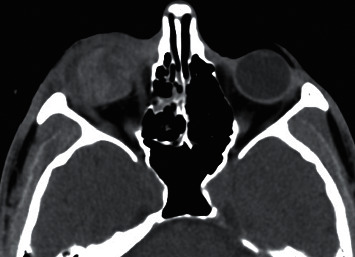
Open globe injury in a 53-year-old woman. Axial unenhanced CT image showing hemorrhage in posterior chamber of the right eye.
